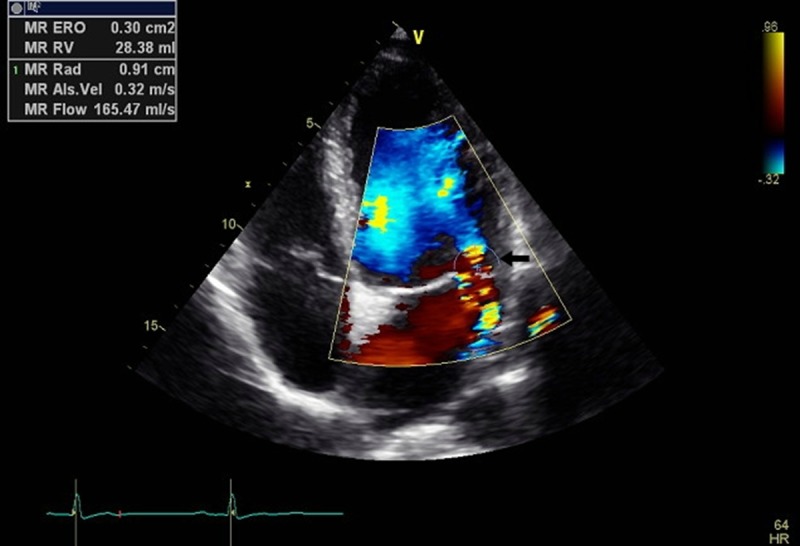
Transthoracic echocardiography showing moderate regurgitation EROA 30 mm^2^, Rvol 28 ml per beat; parameters measured with the help of PISA- standard quantitative assessment of MR
